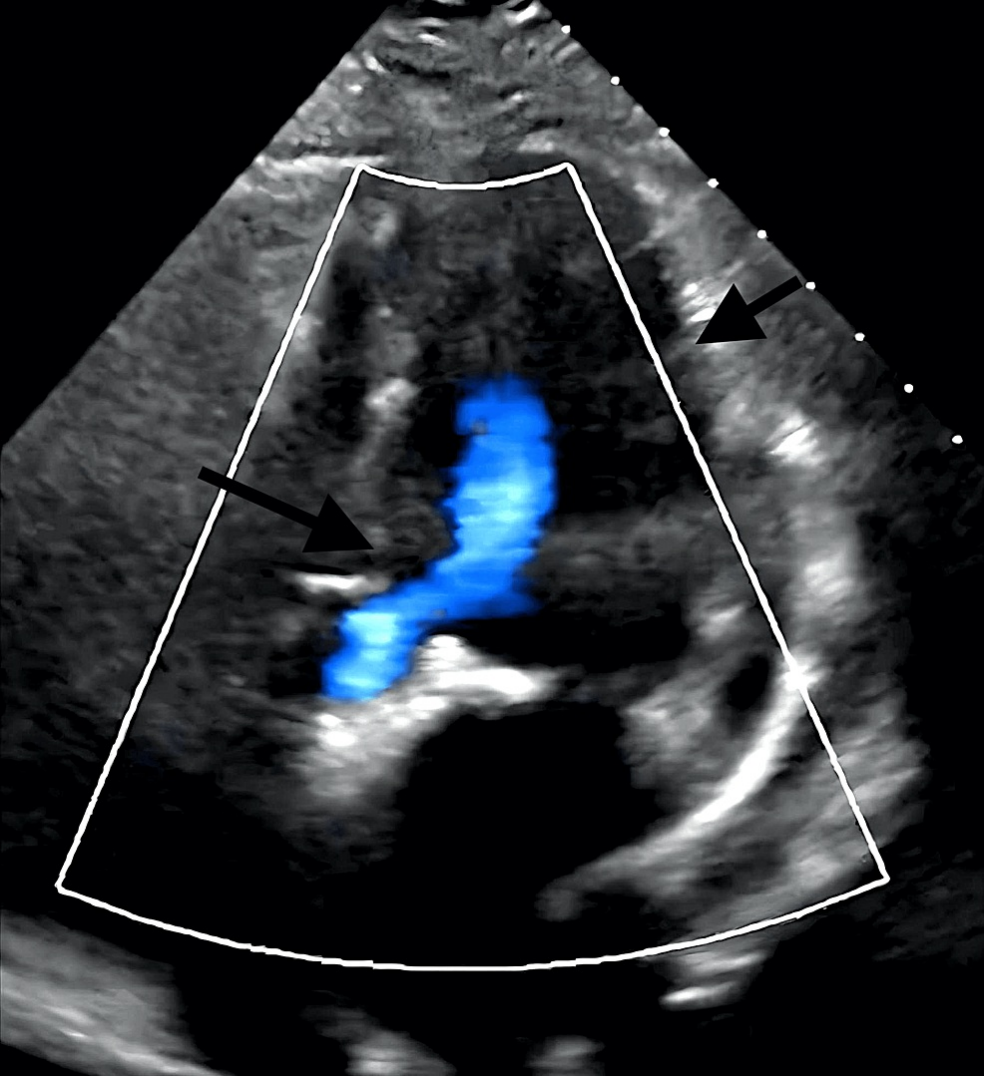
TTE color Doppler after TAVR without evidence of pericardial effusion. There is no post-deployment regurgitation by color Doppler assessment. TTE, transthoracic echocardiography; TAVR, transcatheter aortic valve replacement.
